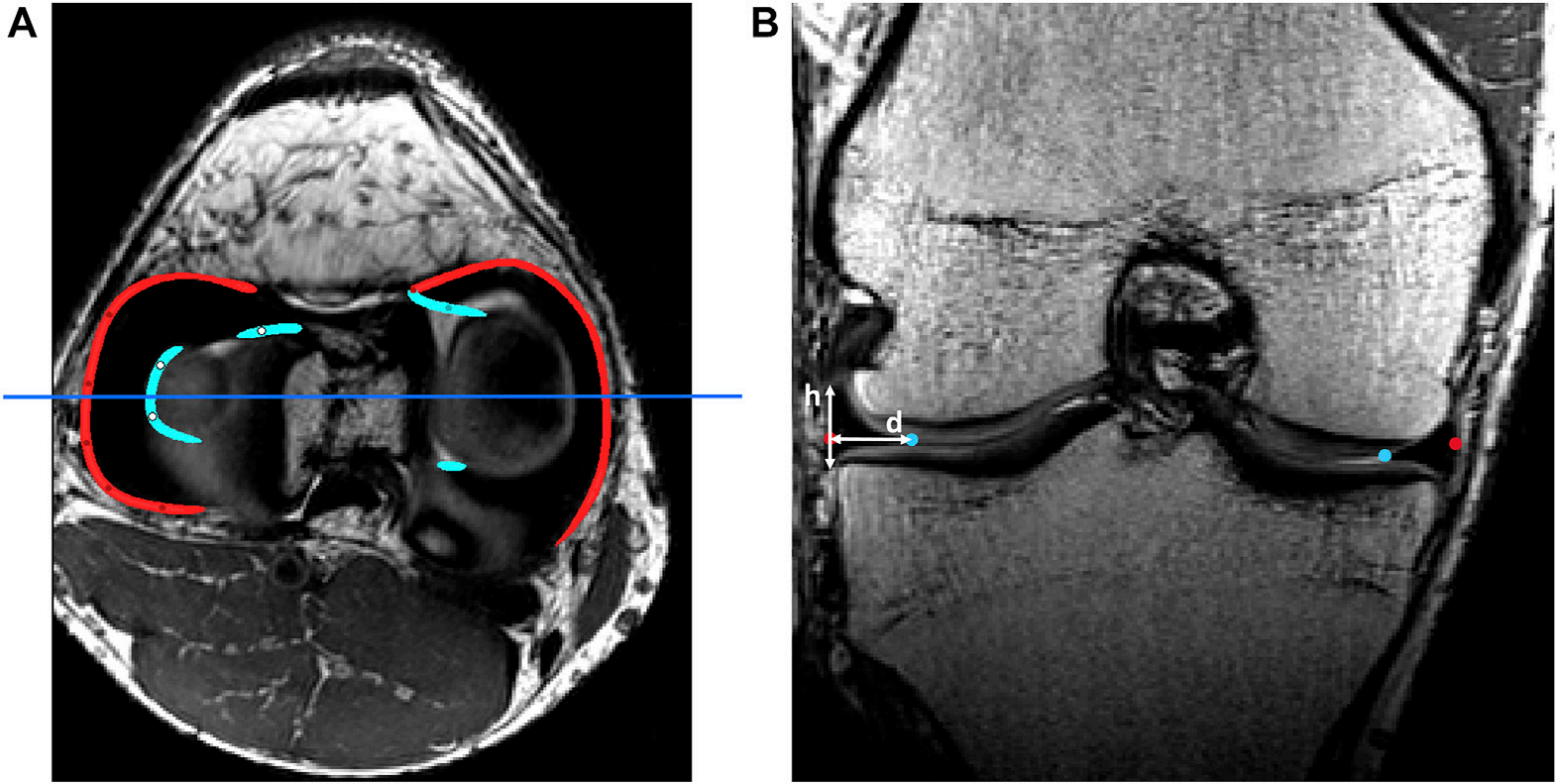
(A) Axial view of the knee joint on MRI with manually detected outer rims (red) and inner rims (light blue). The dark blue line represents the slice of the coronal view. (B) Coronal view of the knee joint on MRI. The height (h) and width (d) of the meniscus were measured as shown. This process was repeated over the course of the outer rim.
